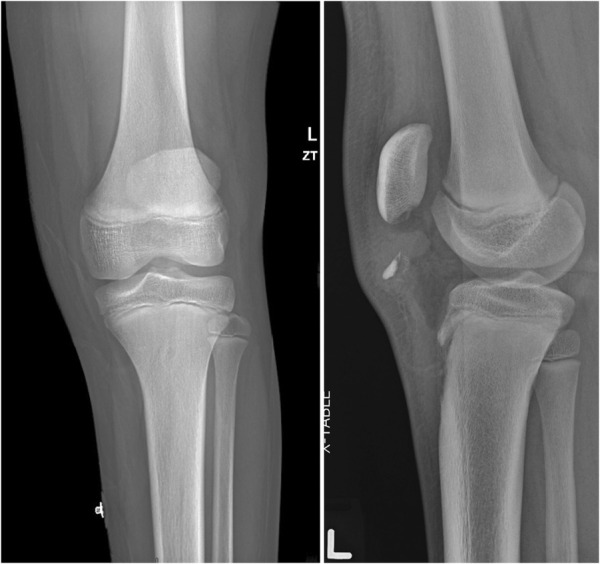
AP and lateral radiographs of the left knee in the Emergency Department.
